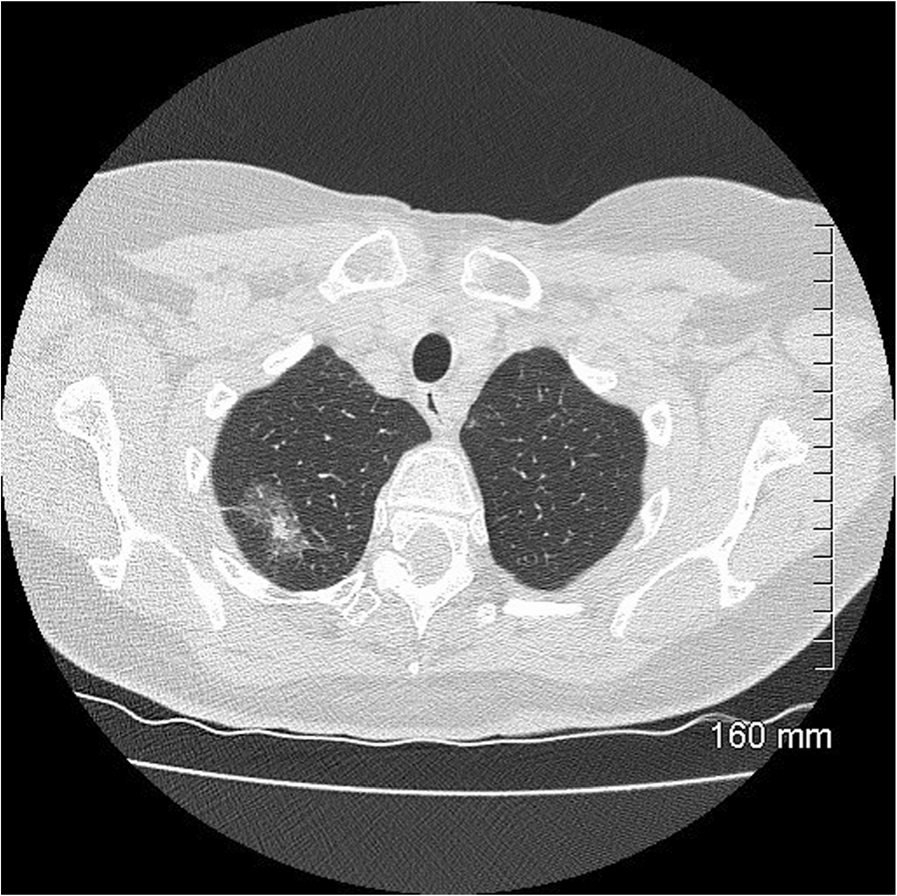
AIS on CT. A 33-mm ground glass opacity in the superior segment of the right upper lobe with a small, central solid component measuring 9 mm × 5 mm
